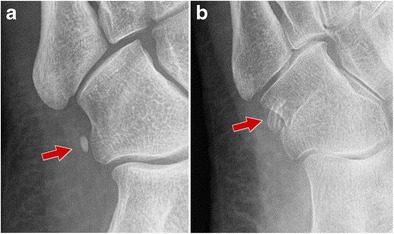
Normal os peroneum - standard radiograph. *2A* Single os peroneum (*arrow*) visualized as an oval, well-corticated ossicle located close to the calcaneocuboid joint. *2B* Bipartite os peroneum (*arrow*): two or more round fragments showing well-defined sclerotic margins
